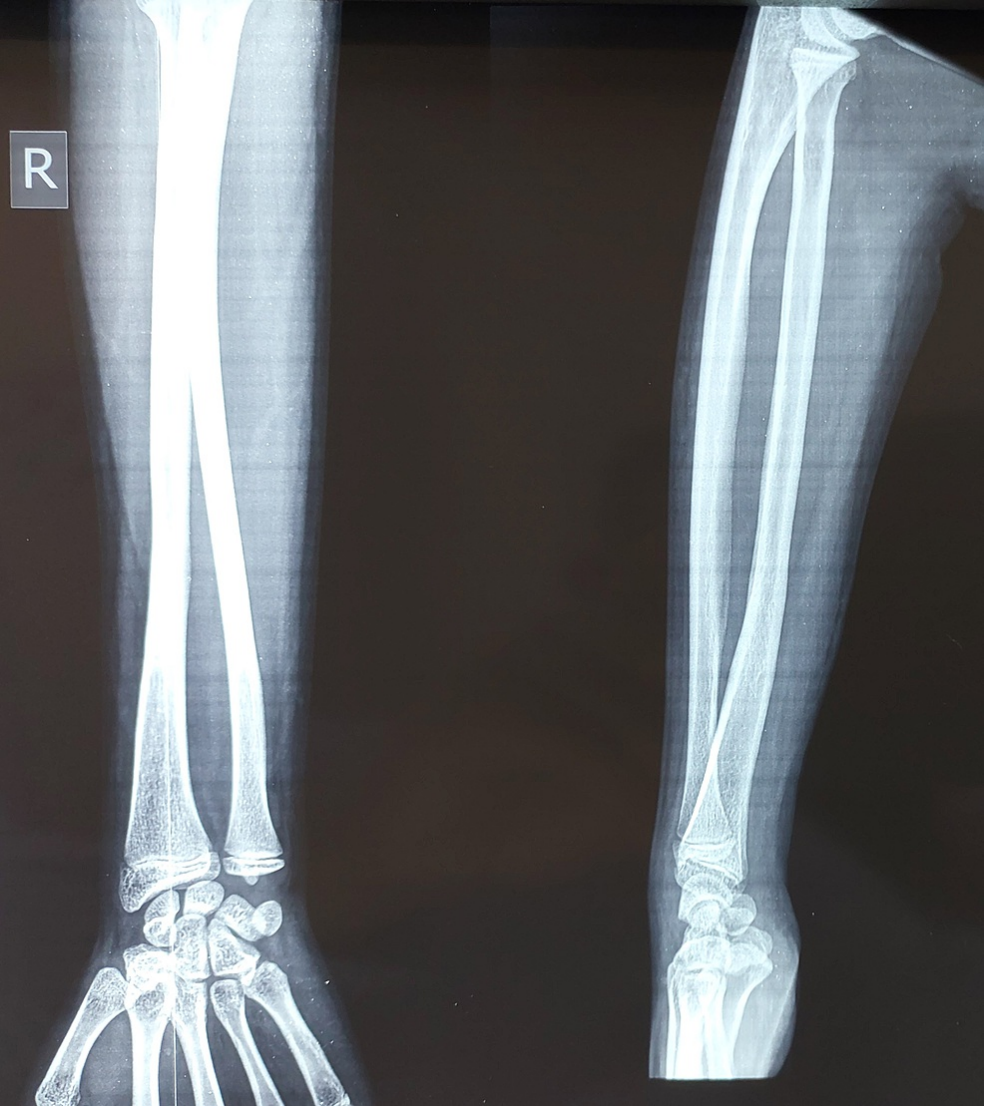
Final check X-ray at the end of four years showing complete radiological healing
